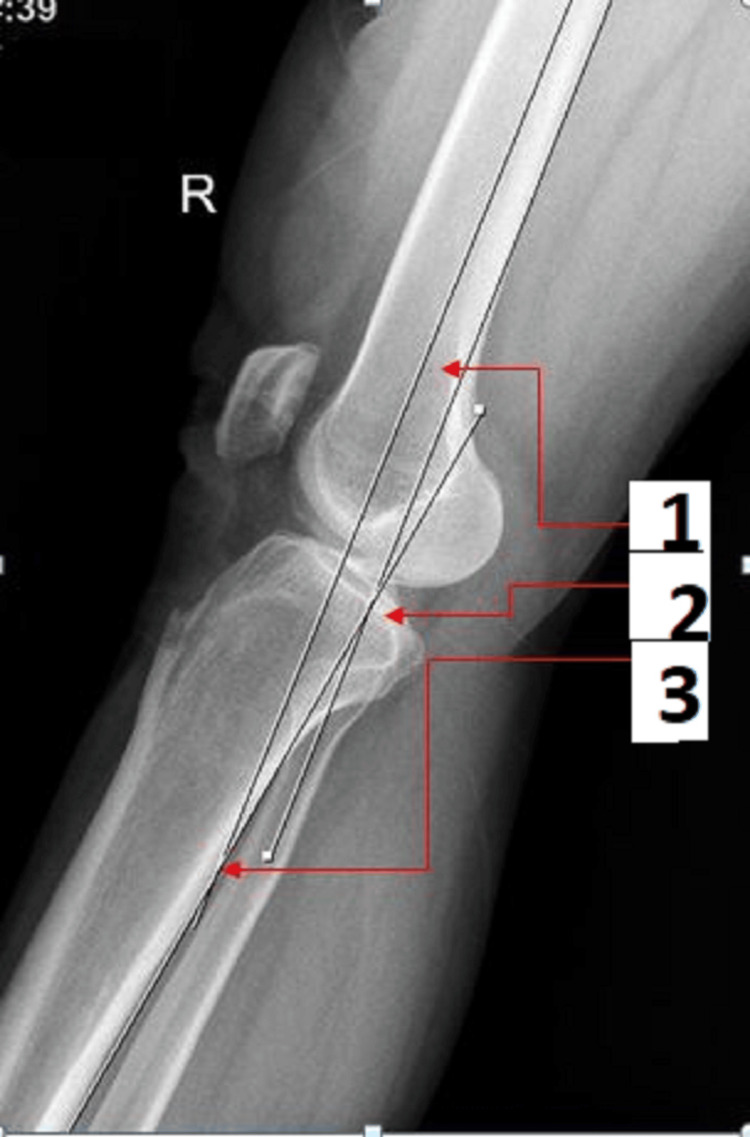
True lateral view X-ray of the knee taken in hyperextension. One line was drawn parallel to the posterior cortex of the femur, and the second line was drawn parallel to the posterior cortex of the tibia. The angle of hyperextension was measured at the point where two lines were transecting each other. 1- A line parallel to the posterior cortex of the femur. 2- Hyperextension angle. 3- A line along the posterior cortex of the tibia.
